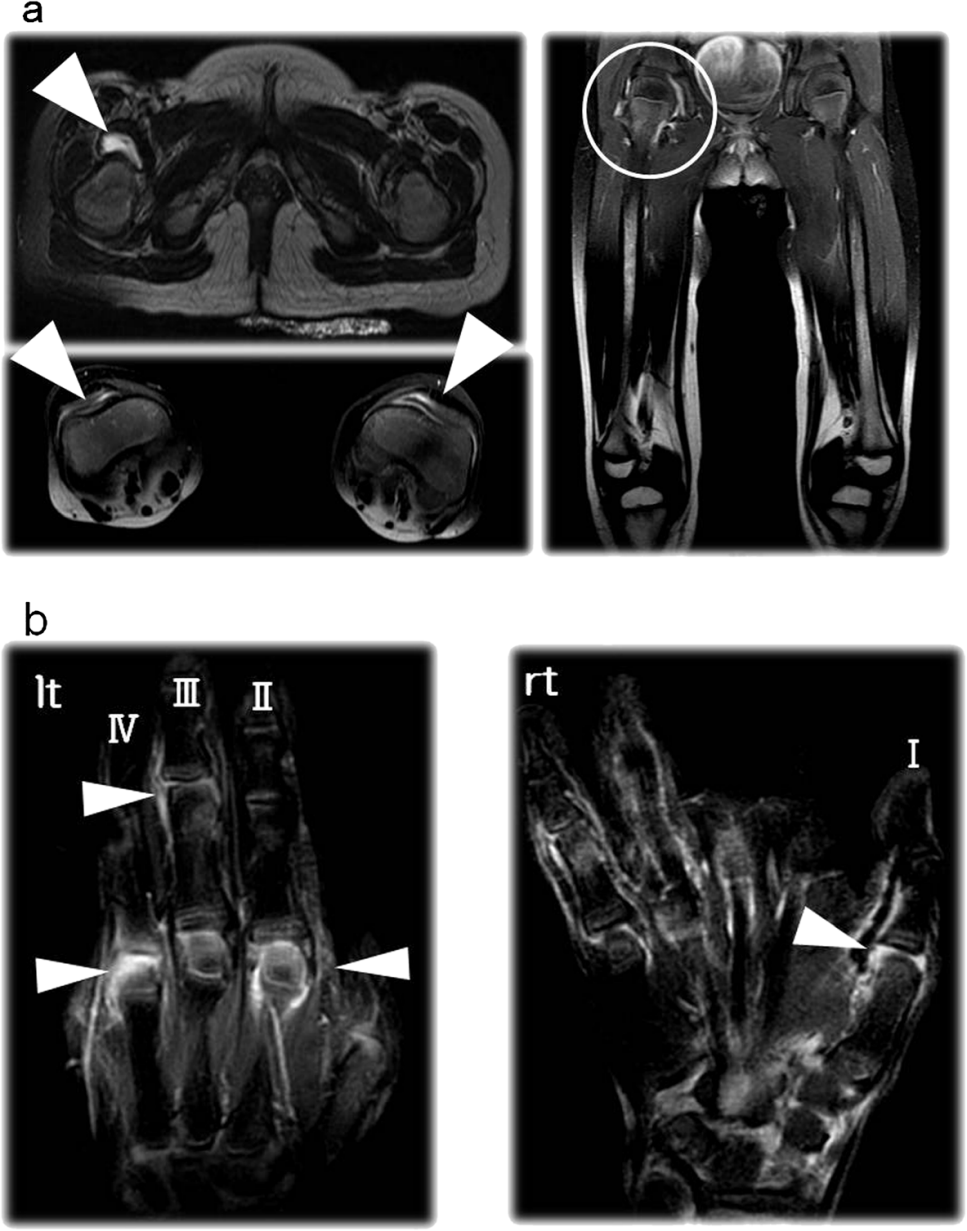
a) MRI of the pelvis, lower extremities and hip and knee joints. Fat SAT T2-weighted image demonstrates chronic inflammation with synovial thickening and/or fluid collection but without bone destruction. b) MRI of hands and fingers. Fat SAT T2-weighted image demonstrates chronic inflammation with synovial thickening and/or fluid collection but without bone destruction.
